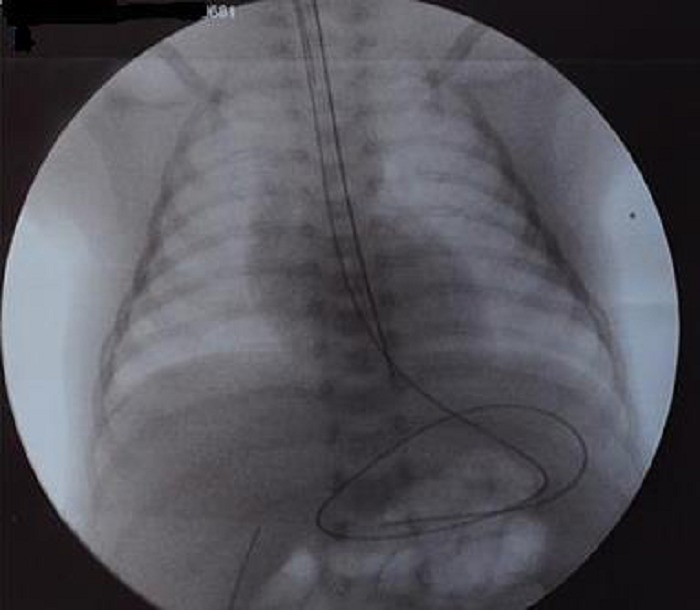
Figure 2: Chest radiograph showing the vascular guidwire which is entering the trachea, then passing through the tracheoesophageal fistula into the esophagus, and ending up curved in the stomach.
